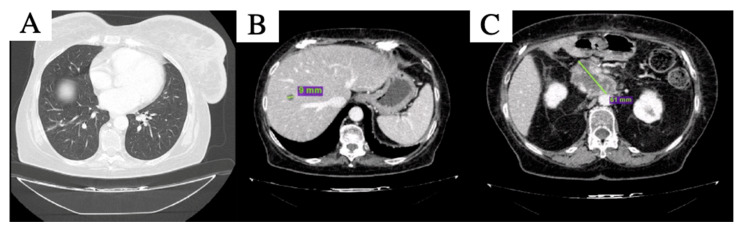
CT scan after 4 months of olaparib: complete disappearance of the nodular lesioni in inferior lobe of the right lung was confirmed (A), the hepatic lesion in the 8th segment (B) is further reduced in size and peripancreatic tissue is slightly increased and substancial stability of the peripancreatic tissue was described (C).
